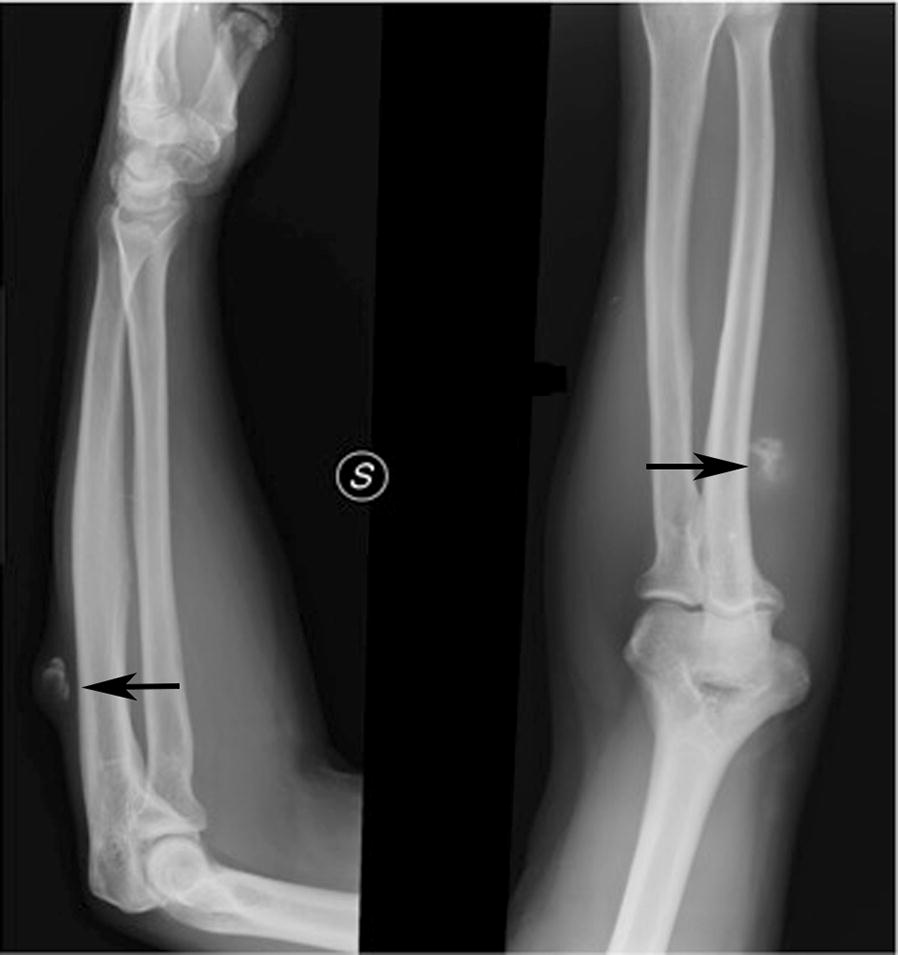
X-ray of the forearm showing a soft tissue tumor with areas of calcification (arrows)
